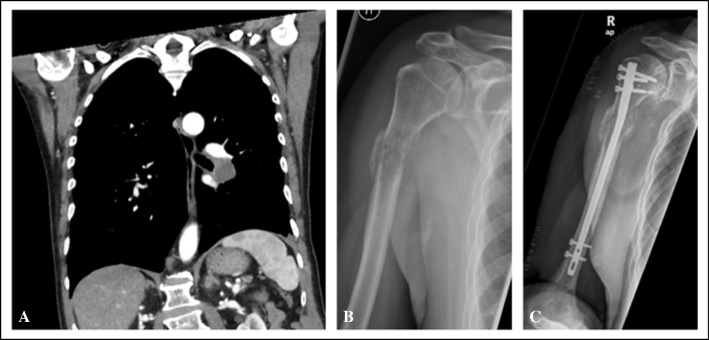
Bone lesion undetected during staging in a 71-year-old patient with lung cancer, presenting with a pathological proximal humerus fracture 5 months post-diagnosis. A Coronal CT of the chest, abdomen, and pelvis was performed 1 month prior for staging, showing the cranial extension of the scan without evidence of the lesion. B Anteroposterior radiograph image of the proximal humerus and shoulder demonstrating a pathological fracture caused by an osteolytic bone lesion. C Postoperative anteroposterior radiograph image of the humerus following closed reduction and fixation with a proximal humerus nail
